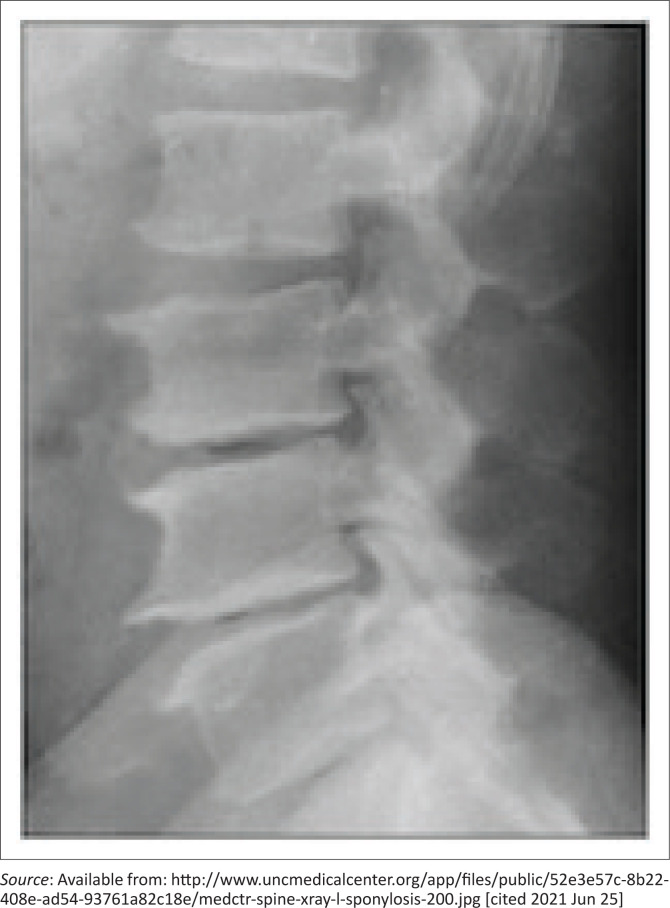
X-ray image for objectively structured clinical examination station.
